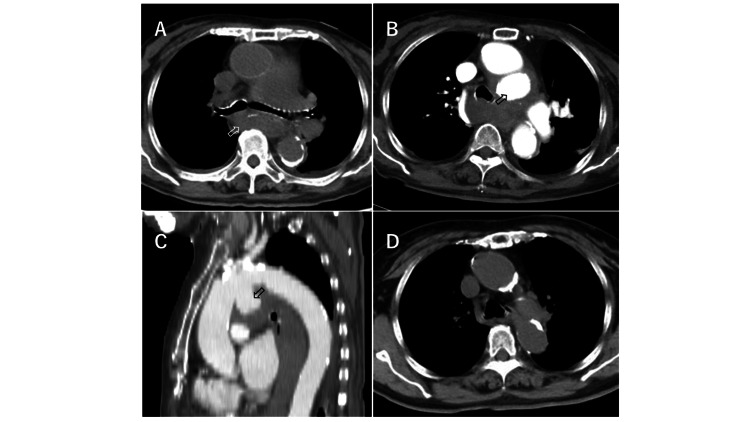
Chest CT after hemodialysis on day 4 Chest plain CT after hemodialysis on day 4, when the patient presented with relapsing severe chest pain, indicated an enlarged mediastinal mass (A; arrow), which turned out to be a hematoma due to the rupture of an aortic arch aneurysm. Finally, the diagnosis of aortic arch rupture was confirmed by contrast-enhanced CT (B, C, arrow, contrast dose: 100 mL). In fact, there was no aortic aneurysm 20 months before the admission (D). CT slice thickness: 5 mm.
